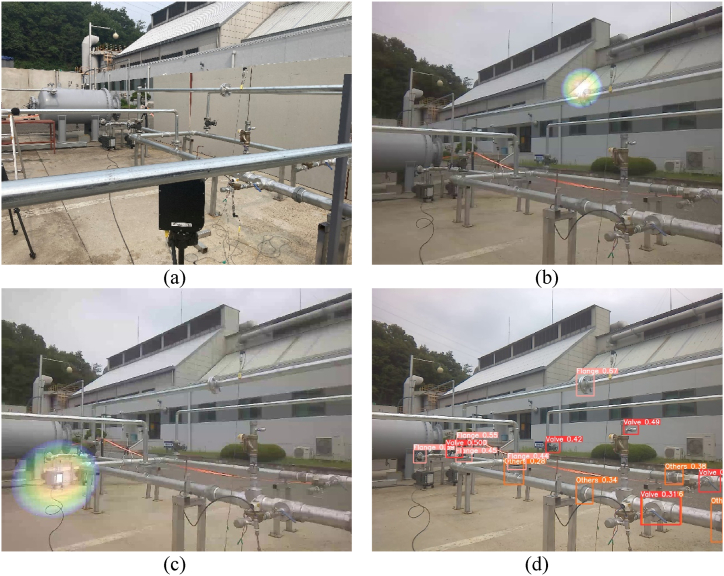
(a) Experiment set-up for case #2. (b) The ultrasonic camera detects the leaky flange without noise. (c) The ultrasonic camera output shows that the gas pump works as a noise source. (d) Pipe joint detection result from the image taken by the ultrasonic camera.
